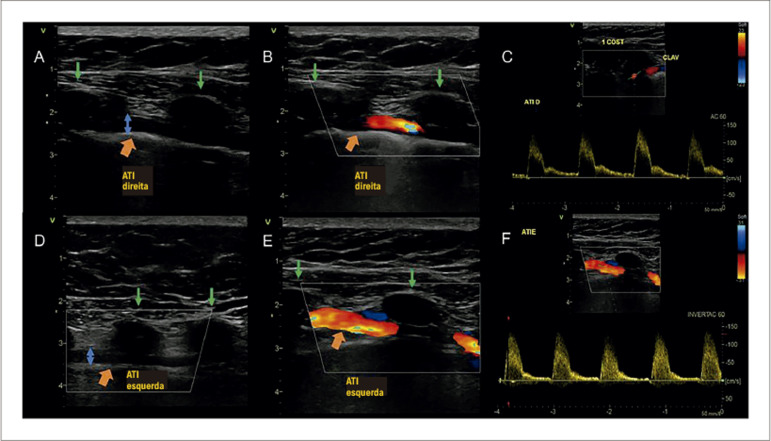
A e D) ATIs direita e esquerda, visualizadas na ultrassonografia bidimensional; B e E) fluxo nas ATI direita e esquerda ao Doppler colorido; C e F) fluxo nas ATIs ao Doppler espectral. Setas laranja: identificar ATIs na ultrassonografia. Setas azuis: medição do diâmetro da ATI direita (3,6mm) e esquerda (3,3mm). Setas verdes: costelas.
